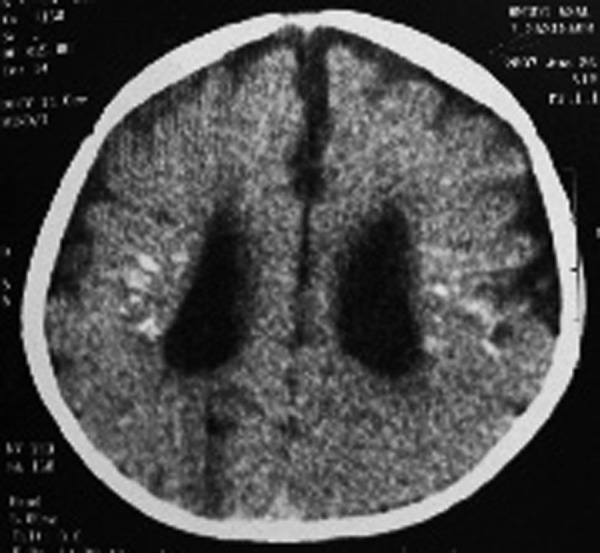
An 18-month-old girl with psychomotor delay. Brain CT scan without contrast reveals mild ventriculomegaly with symmetric punctate and linear periventricular calcifications
